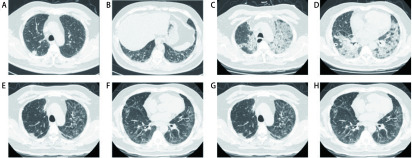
60岁, 男性, IPF合并右肺上叶小细胞癌。A、B：术前胸部CT显示双肺多发网格影、磨玻璃影及索条影, 伴小叶间隔增厚, 以双下肺为著; C、D：术后第3天出现IPF急性加重, 双肺新增多发小叶间隔增厚、磨玻璃影、网格影及索条影, 较术前明显进展; E、F：糖皮质激素治疗14 d后, 双肺弥漫斑片状磨玻璃影较前明显吸收减少; G、H：糖皮质激素治疗42 d后, 双肺间质性炎症吸收消散, 患者顺利出院。 A 60-years-old male, IPF combined with small cell lung cancer in the right upper lobe. A, B: Preoperative chest CT showed multiple reticular shadows, ground glass opacities and strip shadows, accompanied by thickening of interlobular septum, which mainly located in bilateral inferior lobe. C, D: Acute exacerbation of IPF occurred on the 3^rd^ day after surgery. Chest CT showed the newly appeared multiple reticular shadows, ground glass opacities and strip shadows, accompanied by thickening of interlobular septum. The degree of IPF was significantly worse than preoperative lesions. E, F: After 14 days of glucocorticoid treatment, the diffused ground glass opacities in bilateral lungs were significantly absorbed and decreased. G, H: After 42 days of glucocorticoid treatment, bilateral interstitial pneumonia was absorbed and the patient was discharged smoothly.
